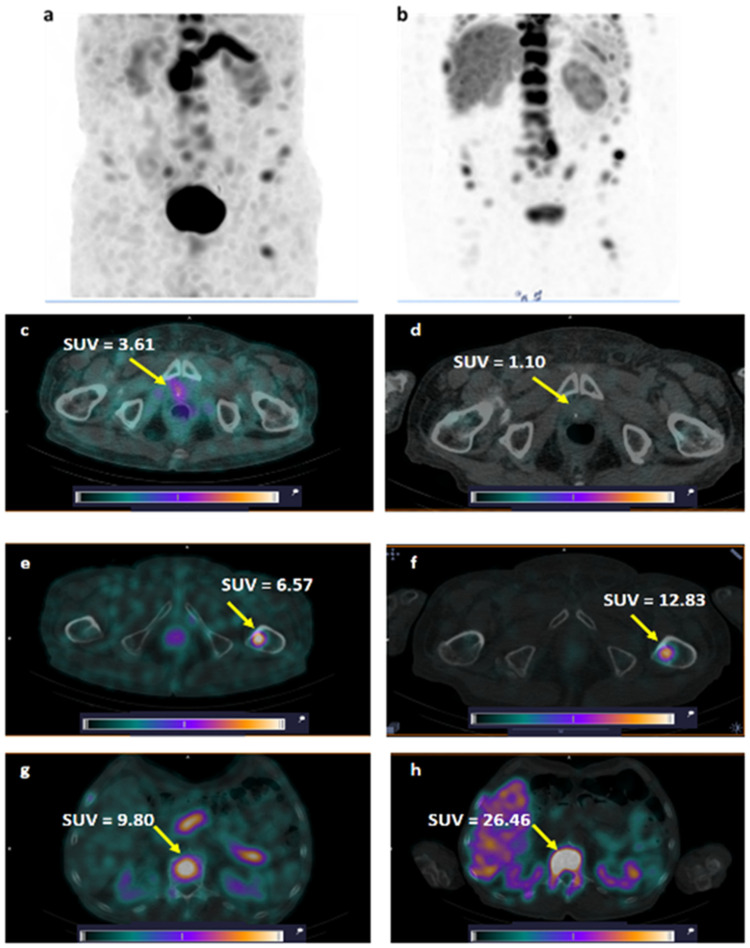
Comparison of visualization of GRPR expression using [^99m^Tc]Tc-DB8 (a) and PSMA expression using [^99m^Tc]Tc-BQ0413 (b) in primary tumor (c,d) and BM (e–h) in Patient 8. A linear relative scale (normalized at the maximum activity in the image SUV_max_ from 0 to 5.0) is applied.
